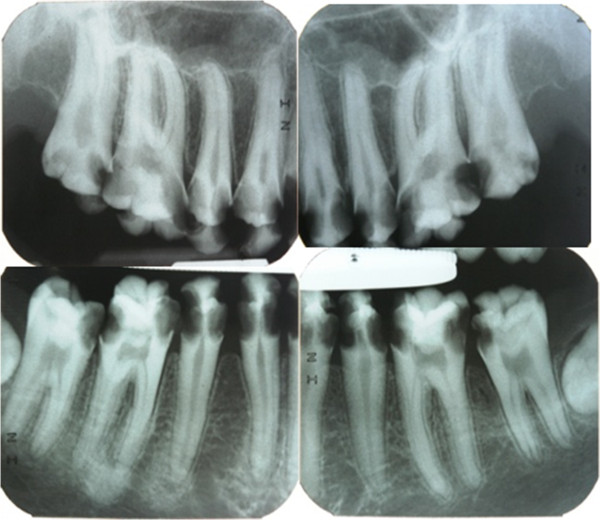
Pre-treatment periapical radiographs.
